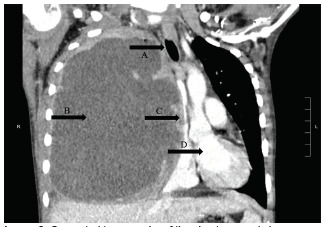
Computed tomography of the chest, coronal view. Massive hemithorax measuring 12.7 × 7.7 × 13.8 centimeters, (B) with leftward mediastinal shift (D) and occlusion of the right mainstem bronchus (A) with significant mass effect onto a patent superior vena cava (C).
